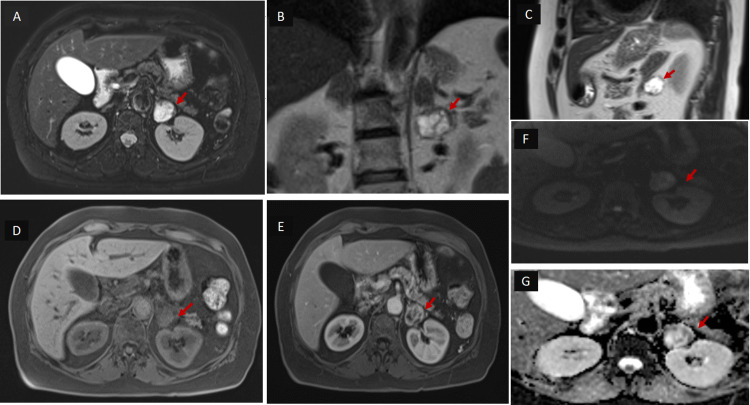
MRI abdomen: exophytic lesion (red arrow) measuring 2.8 x 2.6 cm inferior to the left adrenal gland abutting along the medial cortex of the left kidney with persistent fat planes (A), (B) and (C): Axial fat-saturation, Coronal and Sagittal T2WI: The lesion is hyperintense. (D) Axial T1WI: The lesion is iso to hypointense on T1.  (E) Axial post-contrast: heterogeneous enhancement. (F) and (G): DWI & ADC images: Subtle areas of diffusion restriction. T1W1: T1-weighted image, T2WI: T2-weighted image, DWI: Diffusion-weighted imaging, ADC: apparent diffusion coefficient image
